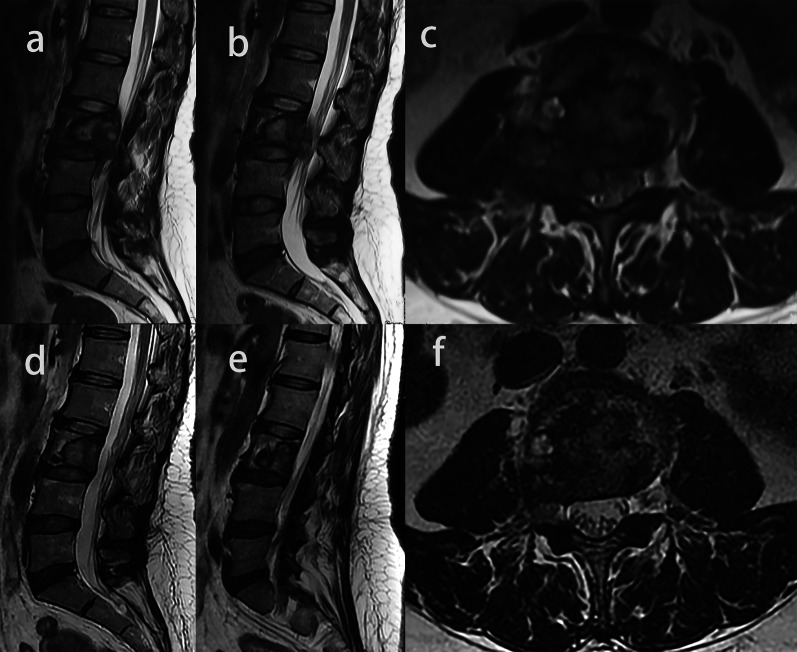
Image of a giant cell tumor of L3 in a 48-year-old woman. a, b, c Axial and sagittal T2-weighted MR images showed epidural lesion before denosumab treatment. d, e, f Axial and sagittal T2-weighted MR images after denosumab treatment. Epidural lesion disappeared completely, and the proportions of the area of the spinal canal occupied by the tumors decreased
